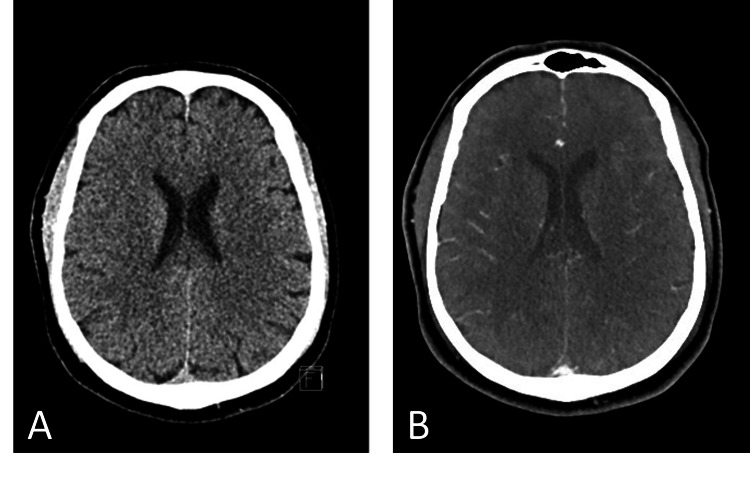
NCCT (A) and CTA (B) images showing no signs of intracranial hemorrhage, brain ischemia or artery occlusion. CTA: CT angiography; NCCT: conventional brain non-contrast computed tomography.
